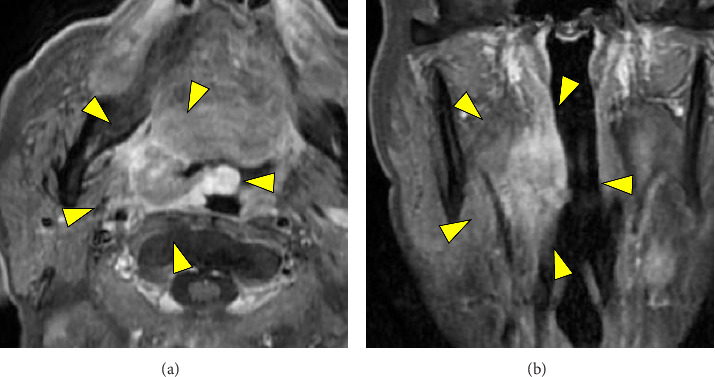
Contrast-enhanced magnetic resonance imaging reveals a mass in the right palatine tonsil (arrowhead). The tumor shows marginal enhancement and invades the surrounding pharyngeal constrictor muscle ((a) axial view; (b) coronal view).
